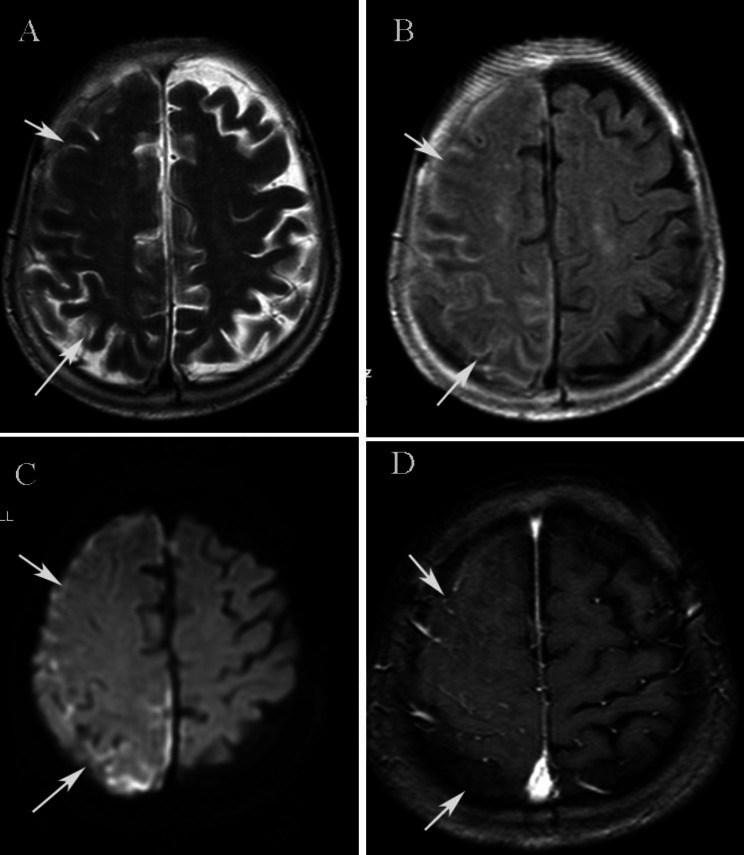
(A). T2-weighted MRI showing decreased signal of the right sulci over the right frontoparietal lobe. (B). T2-FLAIR demonstrating increased signal with obliteration of sulci due to leptomeningeal thickening over the right frontoparietal lobe. (C). DWI showing an increased leptomeningeal signal. (D). Axial post-gadolinium T1-weighted image demonstrating unilateral enhancement of the right leptomeninges
